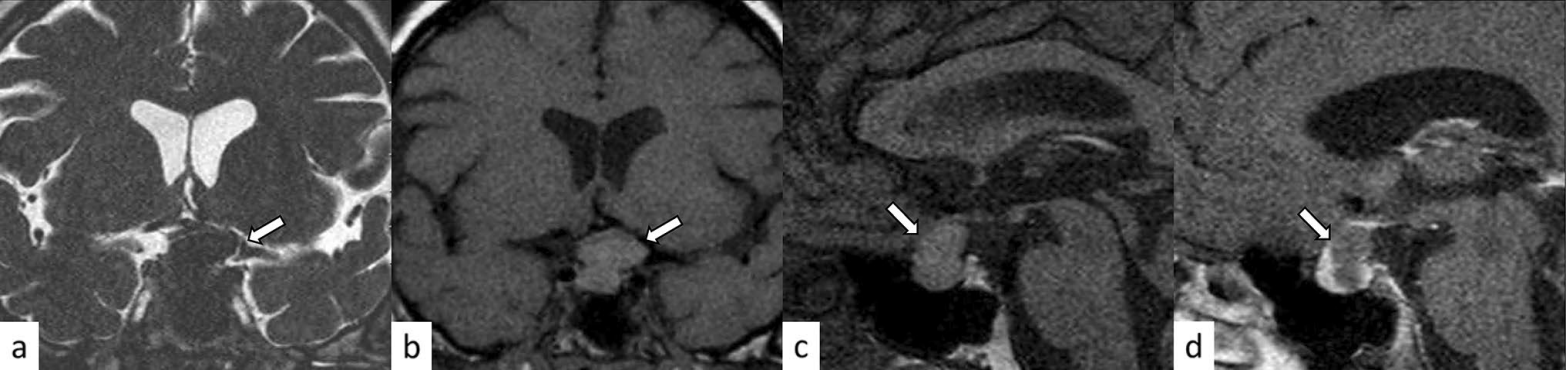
A 24-year-old woman with Rathke’s cleft cyst. There is a sellar/suprasellar mass (a–d, arrows) between the anterior and posterior lobes of the pituitary gland with hypointensity on T2-weighted coronal image (a) and hyperintensity on T1-weighted images b, c without contrast enhancement (d)
